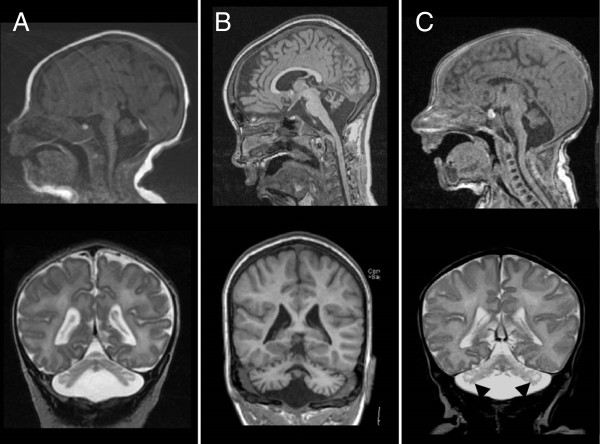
Brain MRI of PCH patients with an *EXOSC3 *mutation. Sagittal and coronal images of a patient with a homozygous p.G31A mutation (A, patient 5-II, age 2w), a patient with a homozygous p.D132A mutation (B, patient 7-I, age 11y) and a patient with a p.D132A mutation and large deletion (C, patient 8, age 1mo). Cerebellar cysts in this last patient are indicated by arrow heads.
